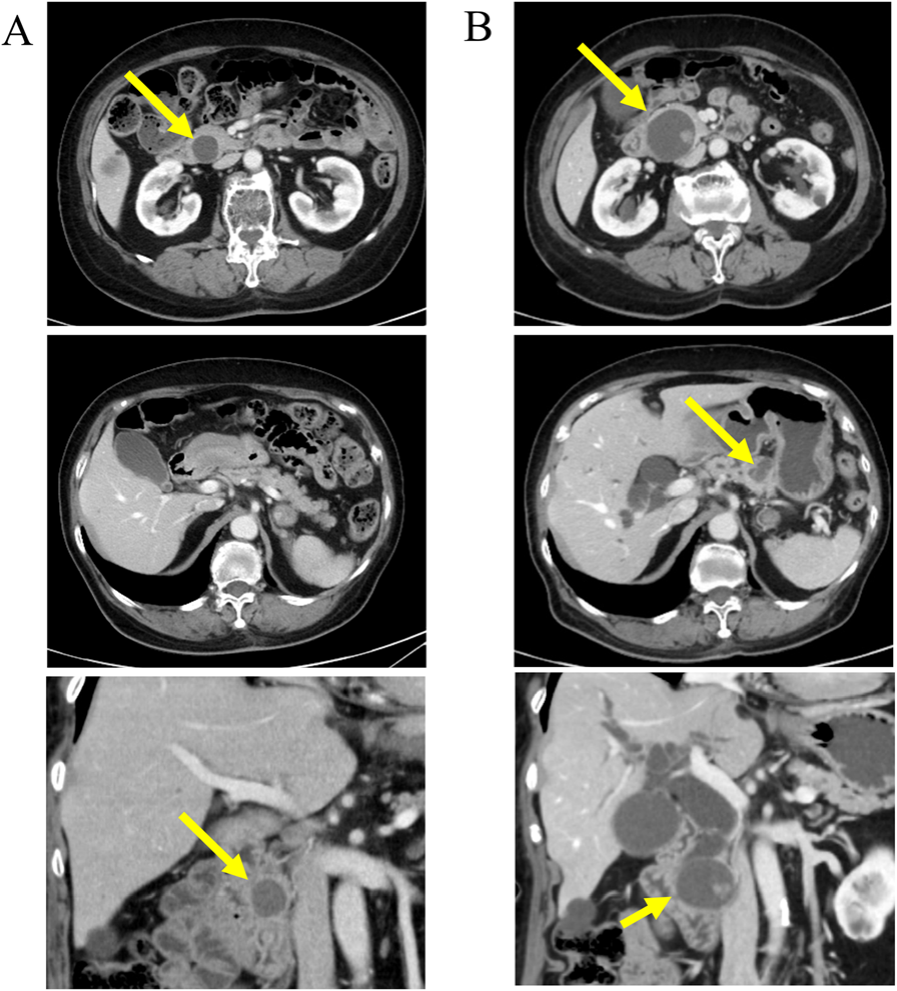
Enhanced computed tomography findings. a CT findings at 1 year after lobectomy for primary lung cancer. A 20-mm cystic tumor was detected on the pancreatic head, with no tumor on the pancreatic body. b CT findings at 2 year after lobectomy. The cystic tumor on the pancreatic head gradually increased from 20 mm to 37 mm and showed a contrasted solid nodule inside the cystic tumor. The cystic tumor was also indicated at the pancreatic body
